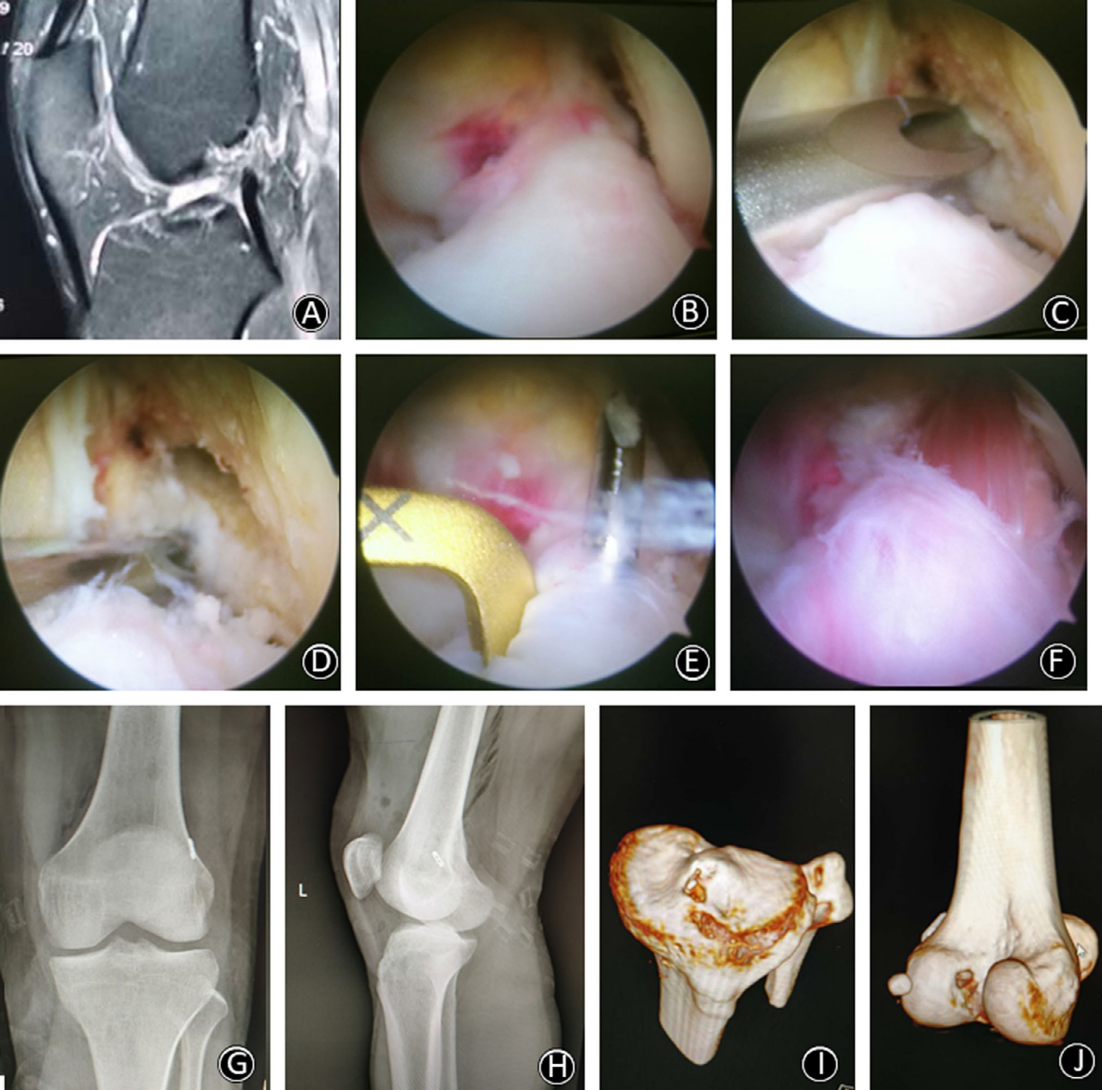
(A) MRI showed that ACL was broken and formed a low flat shape in the joint. (B) Arthroscopic rupture of ACL was observed, and the scar on posterior cruciate ligament (PCL) had healed. (C) After marking the I.D.E.A.L. point with a plasma knife, the Kirschner wire was positioned through the ACL femoral locator for femoral drilling. (D) Intraoperative check of the position of the thick femoral bone tunnel. (E) A tibial drill guide was inserted through a standard anteromedial portal and positioned at the center of the remaining ACL footprint. (F) The graft was fixed through the tibial and femoral bone tunnel in order to observe it under arthroscopy. It was found that the graft just passed the center of the ACL stump and the surface was covered by the fibers of ACL remnant. (G,H) Postoperative anteroposterior and lateral plain radiographs showed the correct position of the TightRope button. (I) 3D CT scan reconstruction with a tibial tunnel position. (J) 3D CT scan reconstruction with a femoral tunnel position.
